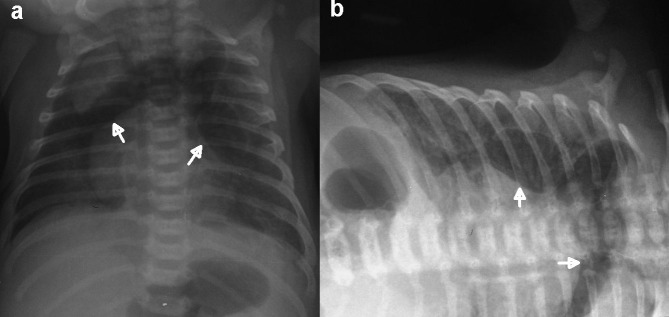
Figure 1: Chest X-ray films: (a) supine position (b) cross-table lateral view. Chest X-ray films demonstrate multiloculated large quantity of air in the mediastinum (white arrows), causing elevation of the thymus. There is no displacement of the multiseptated- multiloculated air on cross table lateral view.
